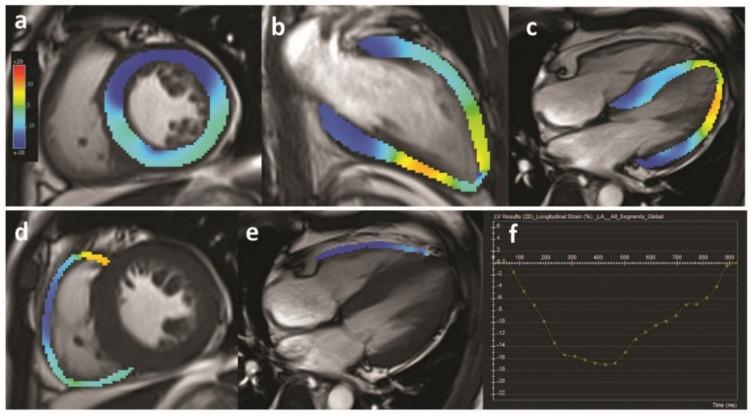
Example of myocardial strain analysis using MRI in a patient with CKD. Quantification of left ventricular strain (a–c) and right ventricular strain (d,e) parameter is visualized by colored overlay on cine images. (f) is an example of strain–time curve of the left ventricular global longitudinal strain within one cardiac cycle.
